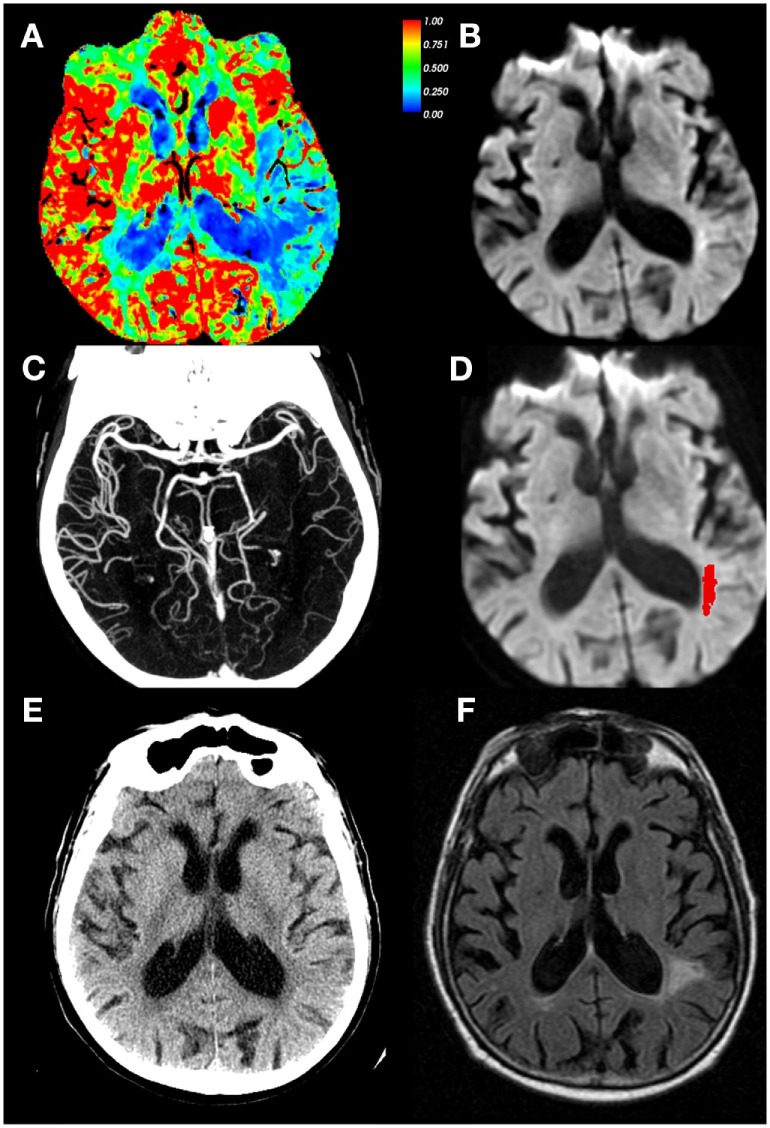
CT perfusion (CTP) cerebral blood flow (CBF) maps do not correspond with infarct core. Eighty-four-year-old male with left middle cerebral artery (MCA) stroke with dense distal M2 occlusion presenting with initial National Institutes of Health Stroke Scale (NIHSS) of 9. By the time of the admission, NIHSS was 2 and patient did not receive IV tPA or endovascular treatment. The CTA/ CT perfusion (CTP) was acquired at 4.9 h from last-known well (LKW), magnetic resonance imaging was performed 19 min after the CTP. (A) CBF, (B) diffusion-weighted imaging (DWI) performed 19 min after CTP, (C) CTA shows occlusion of MCA superior division segment, (D) DWI with acute infarct mapped in red, (E) CT head at 24 h from LKW, and (F) follow-up FLAIR image at 34 days post-stroke depicting final infarct. Note the CTP CBF hypoperfused region identified as 30% of mean contralateral hemisphere is much larger than that of the DWI scan, and corresponded better with tissue infarction on follow-up. *Courtesy of William A. Copen, MD, Department of Radiology, Massachusetts General Hospital. Data analysis for figure was created under approval of local ethics committee*.
